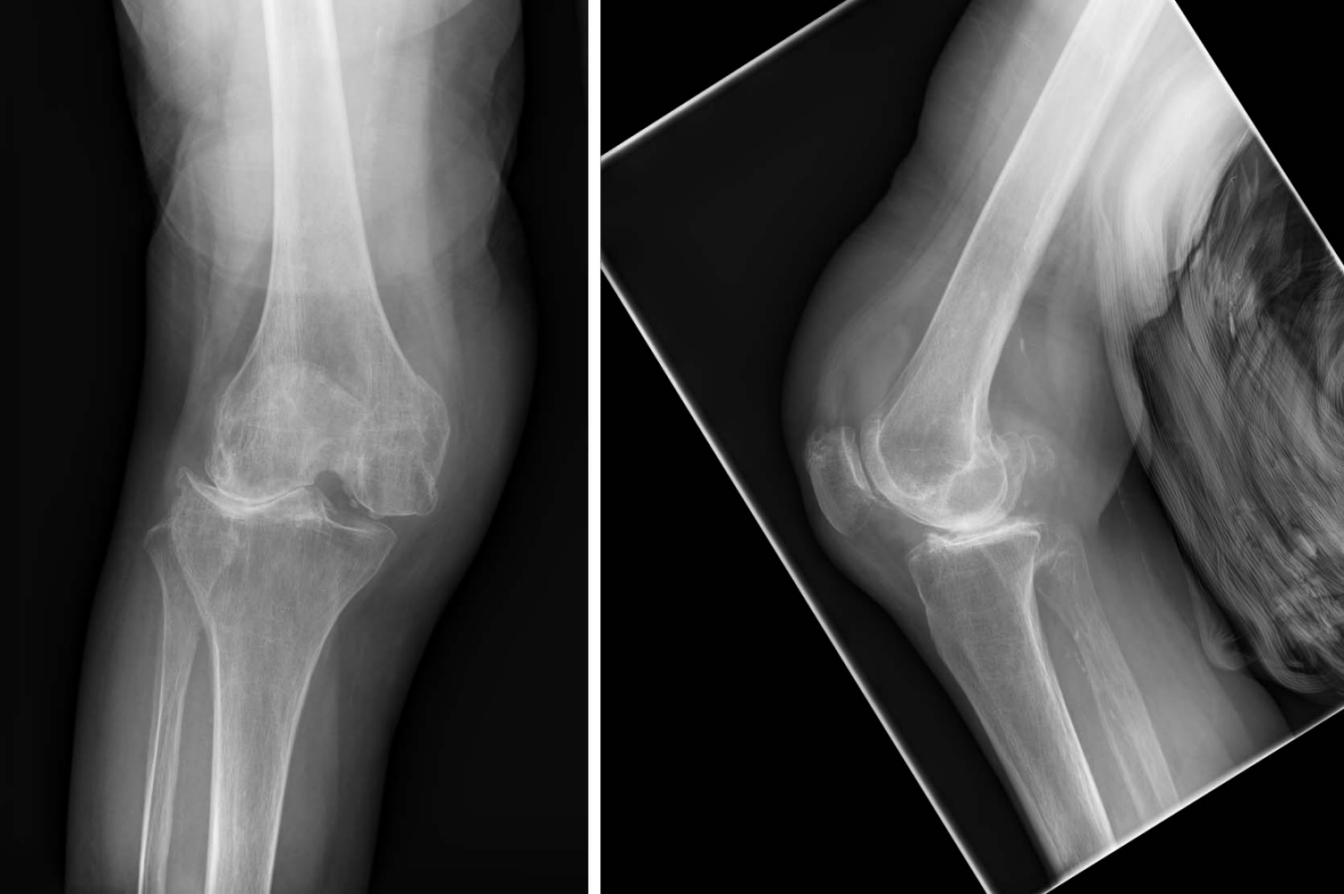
Preoperative X-ray of the right knee joint Fracture of the intercondylar fossa of the distal femur. No fracture of the tibia. No fracture of the fibula. Severe gonarthrosis. Laterally, the joint space is narrowed with pronounced subchondral marginal sclerosis and osteophytes. Subluxation position.
